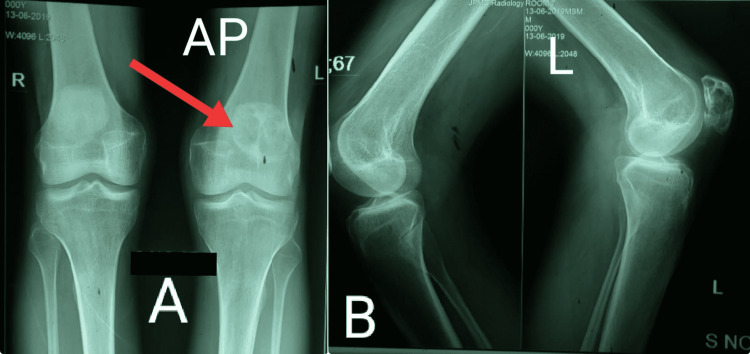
Antero-posterior (A) and lateral bilateral (B) knee joint radiography clearly demarcating the enlarged lytic lesion in the left patellar bone.
